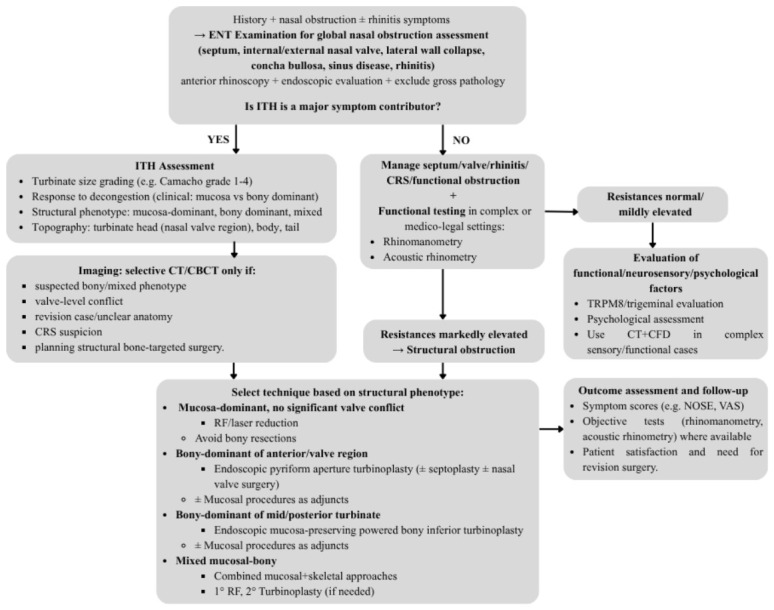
Phenotype-based algorithm for the management of inferior turbinate hypertrophy within the broader work-up of nasal obstruction. The algorithm integrates endoscopic assessment, functional testing and structural phenotyping (mucosal, bony or mixed hypertrophy; anterior nasal valve involvement; turbinate head/body/tail), and links each structural phenotype to a preferential surgical strategy (mucosa-preserving techniques, pyriform aperture turbinoplasty, endoscopic mucosa-preserving powered bony inferior turbinoplasty or combined approaches). Functional testing (rhinomanometry, acoustic rhinometry and, when available, PNIF) and, in selected cases, CT/CBCT with or without CFD modelling, as well as trigeminal/TRPM8 and psychological evaluation, are used to differentiate true structural obstruction from predominantly functional, neurosensory or psychogenic nasal obstruction and to avoid mismatched treatments. ITH: inferior turbinate hypertrophy; RF: radiofrequency; CT: computed tomography; CFD: computational fluid dynamics; NOSE: Nasal Obstruction Symptom Evaluation; VAS: visual analogue scale; TRPM8: transient receptor potential melastatin 8.
